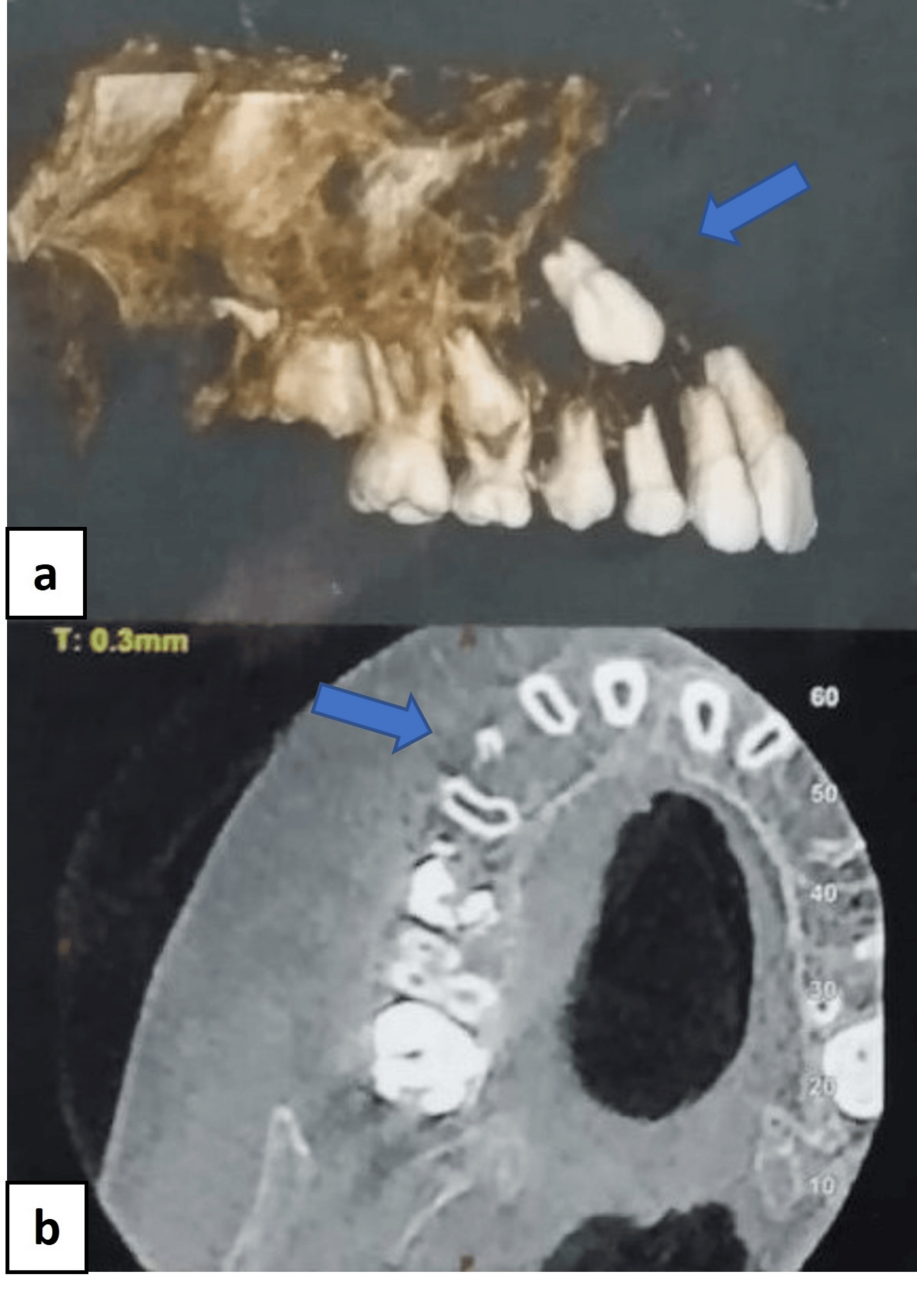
(a) Cone-beam computed tomography reconstructed image showing bone destruction in the right maxillary region. (b) Axial view showing an ill-defined, hypodense osteolytic lesion with irregular borders and periphery.
